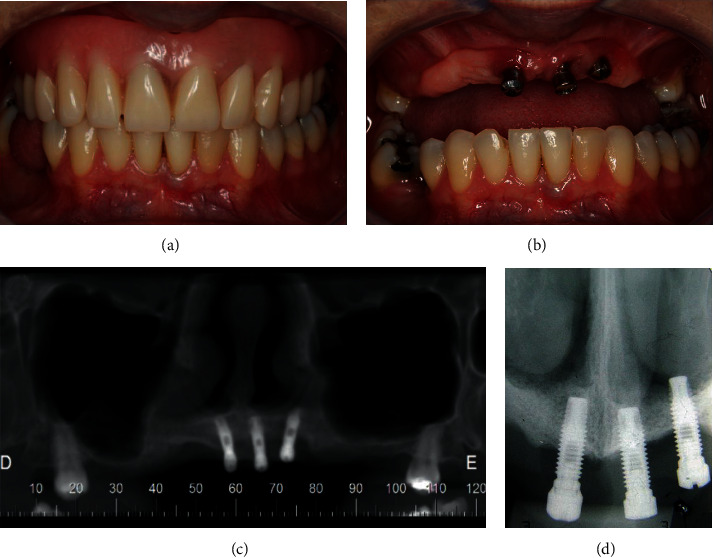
Intraoral images of the prosthesis in placement (a). After prosthesis removal, it is possible to observe gingival inflammation and swelling of the implants (b). Panoramic and periapical radiographies show evident bone resorption around the implant threads and severe pneumatization of the maxillary sinuses (c, d).
